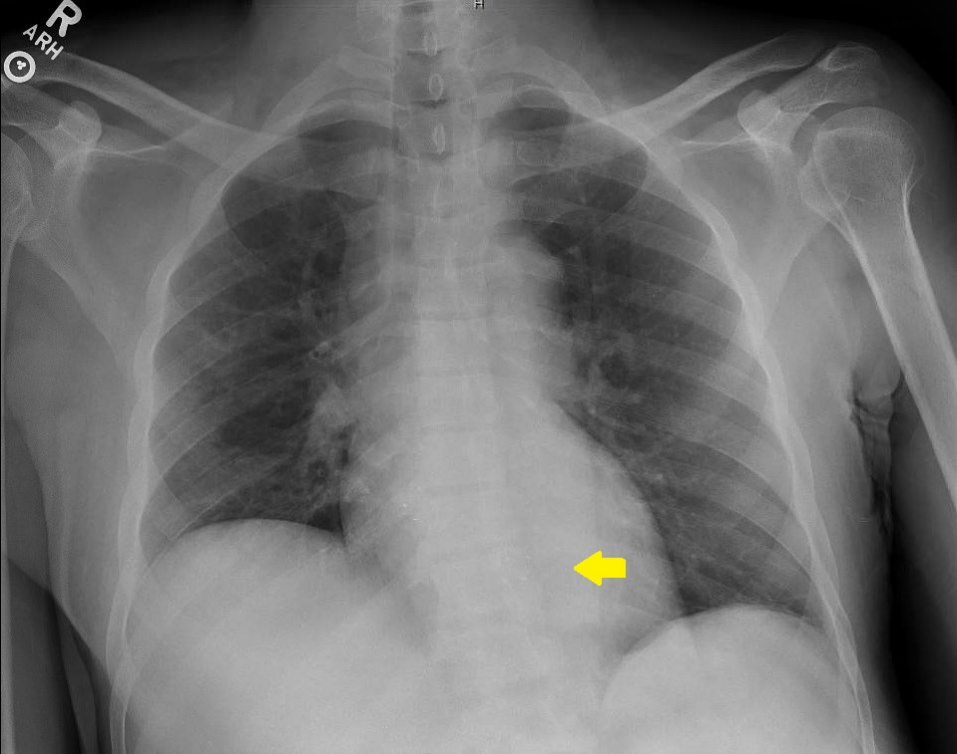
Anteroposterior chest x-ray showing punctate intracardiac markings.
